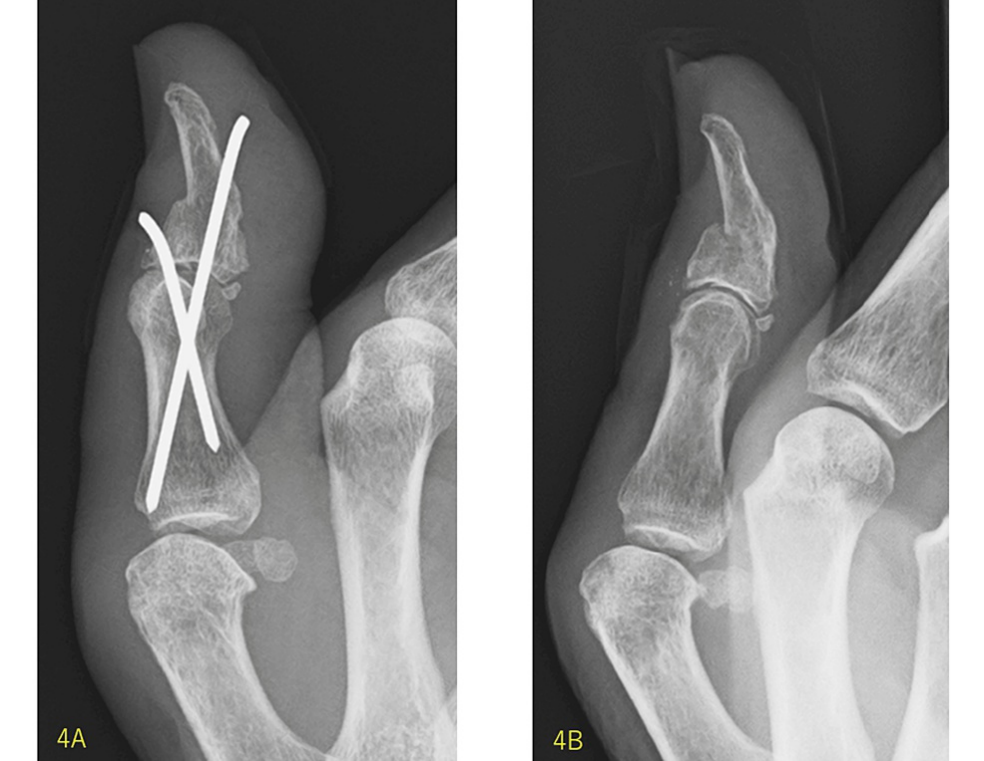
X-ray radiography after the operation. The Ishiguro extension block technique was carried out with two 1.5 mm K-wires. The fracture fragment was stabilized well. The K-wires were cut as short as possible and buried under the skin (4A). In the X-ray radiography six weeks after the operation, the K-wires were removed, and the bone union was good (4B).
